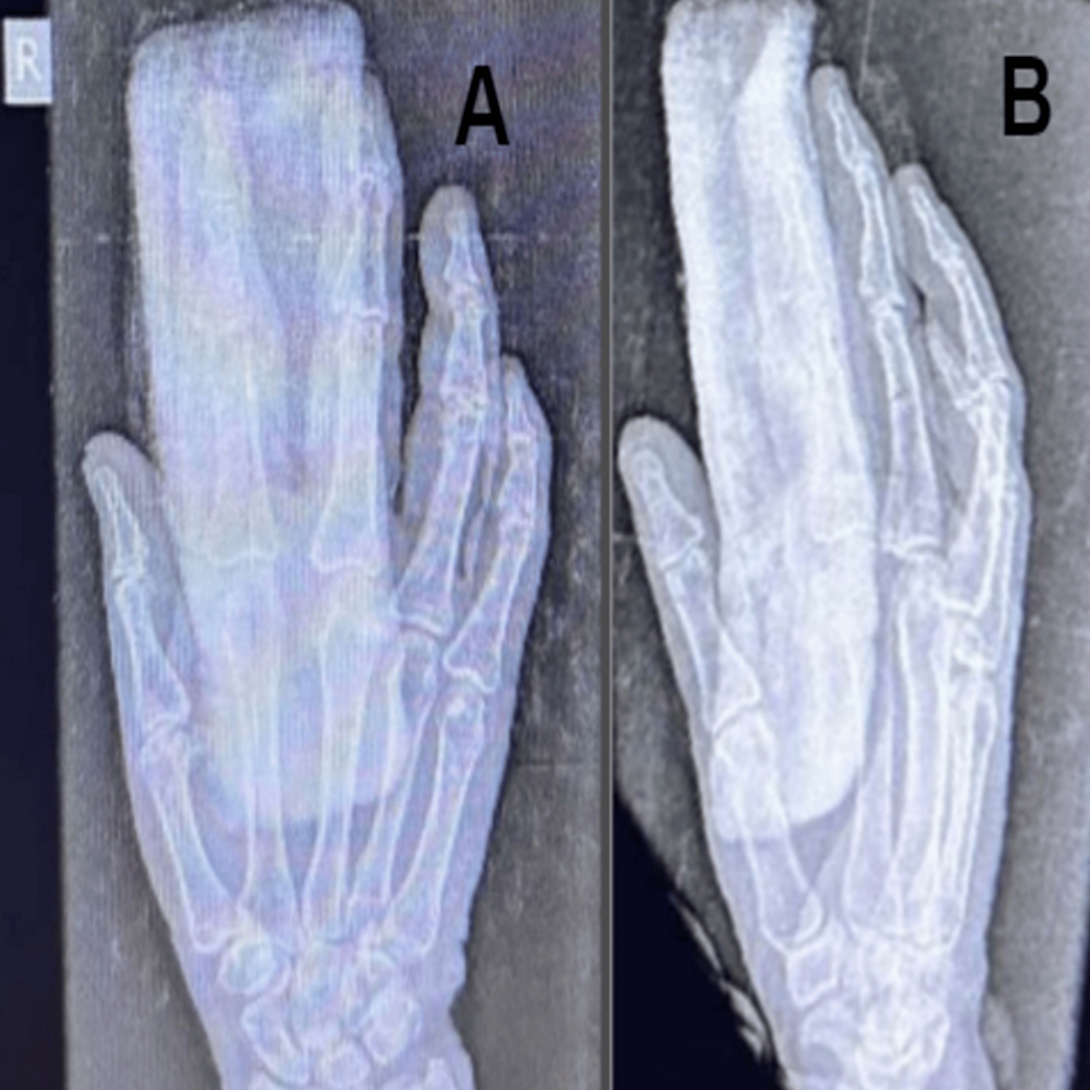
Post-op X-ray of the right hand AP and lateral view 5A: Post-op X-ray of the right hand showing the AP view; 5B: Post-op X-ray of the right hand showing the lateral view.
